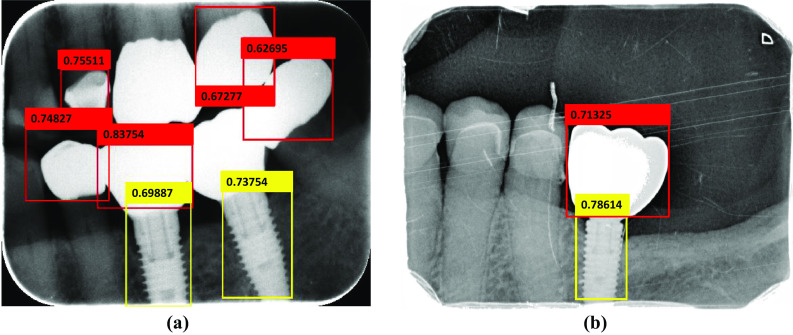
Examples of intraoral radiographs with implants in the lower jaw
